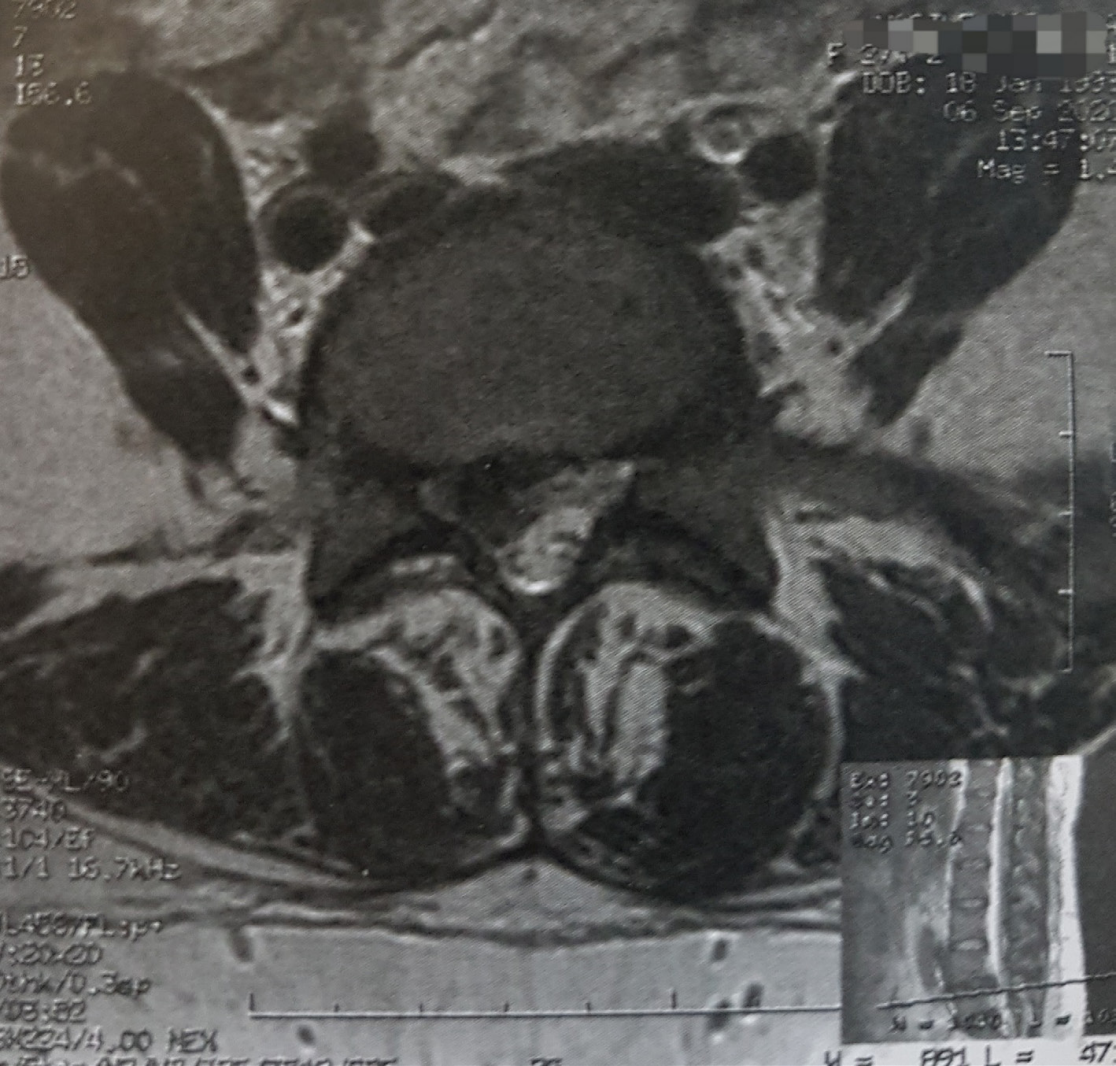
T2-weighted MRI scan, axial section, showing a caudally-migrated L4/L5 disc fragment.
